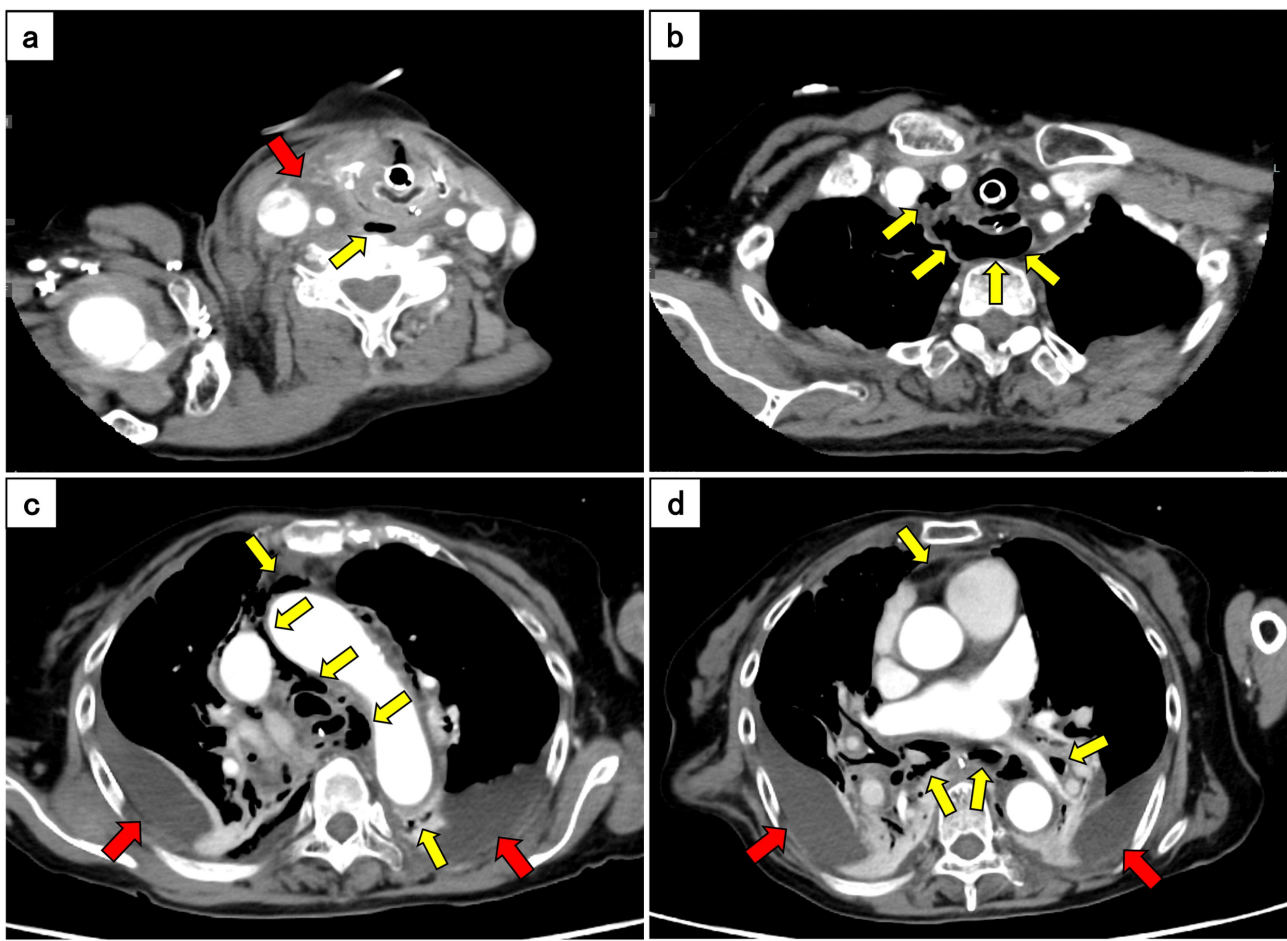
Contrast-enhanced computed tomography on admission in the axial plane (a) Low-density areas in the carotid and retropharyngeal spaces (red arrow) with air tracking extending from the retropharyngeal space to the mediastinum. (b-d) Extensive pneumomediastinum (yellow arrows) with bilateral encapsulated pleural effusion (red arrows).
